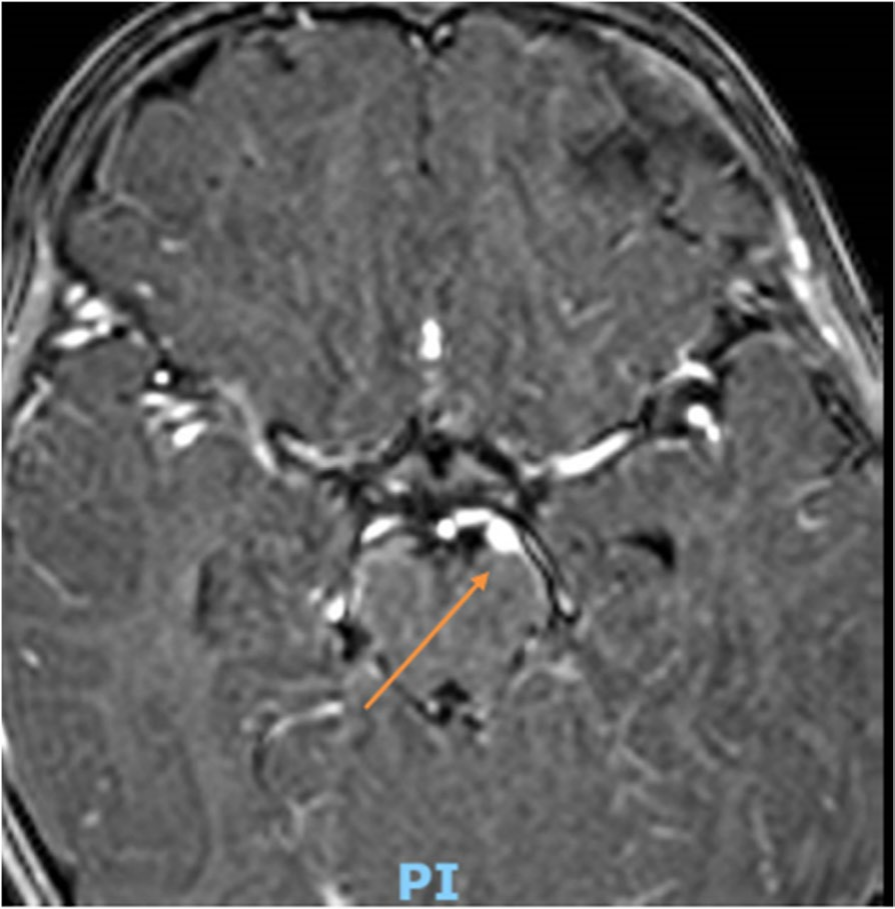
First MRI wrongly suggestive for an aneurism along the medial side of the left cerebral peduncle
